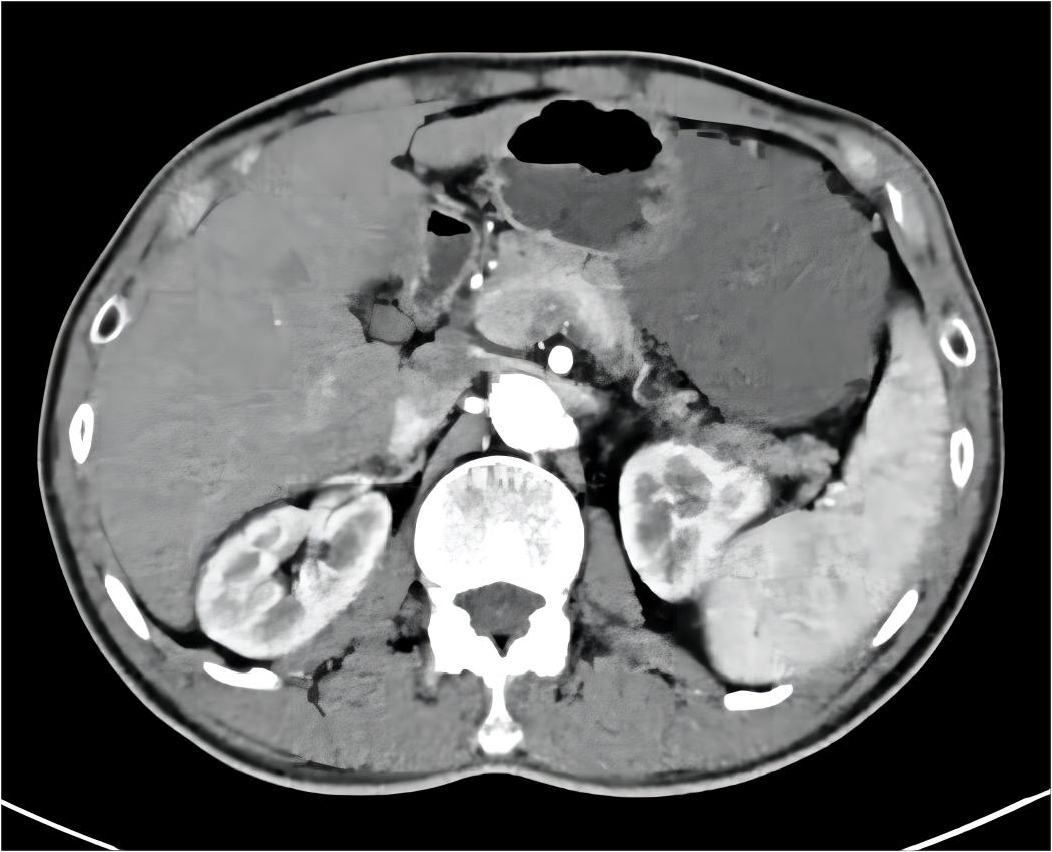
Post-treatment abdominal CT results with imatinib: the primary tumor is significantly reduced.
